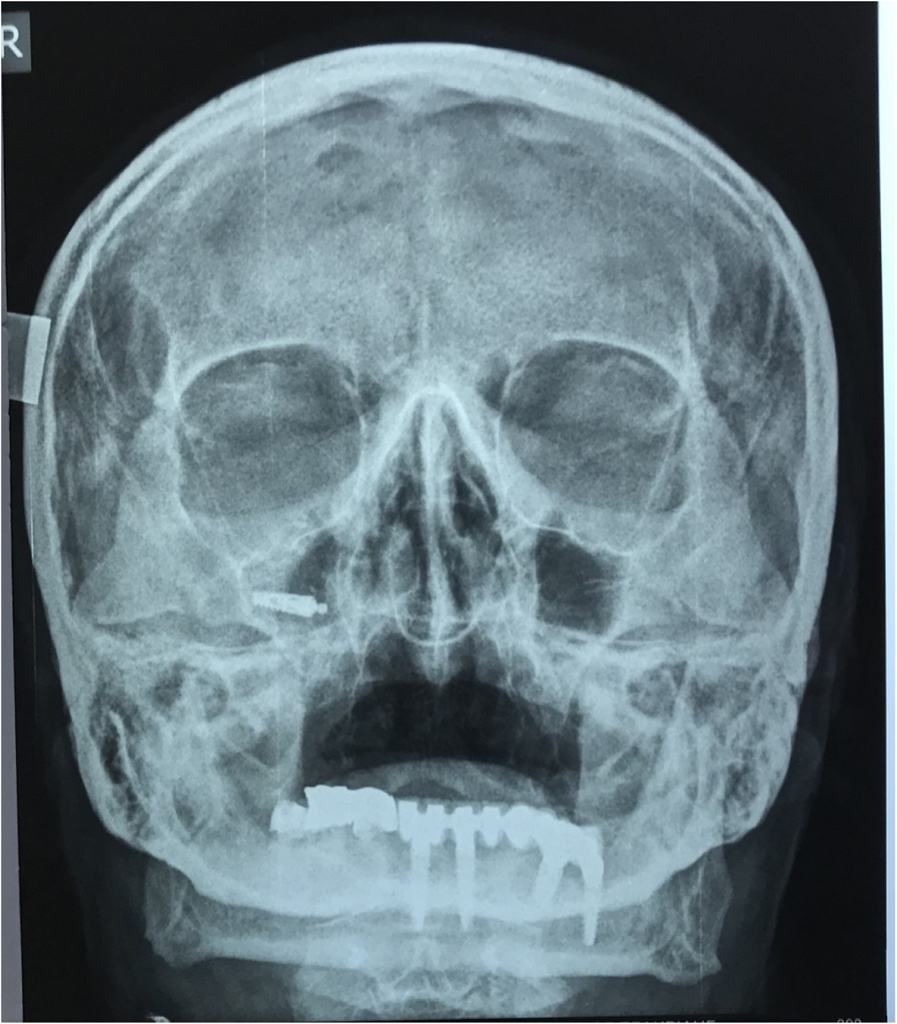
Water’s X-ray which confirmed the migrated implant into the right maxillary sinus
